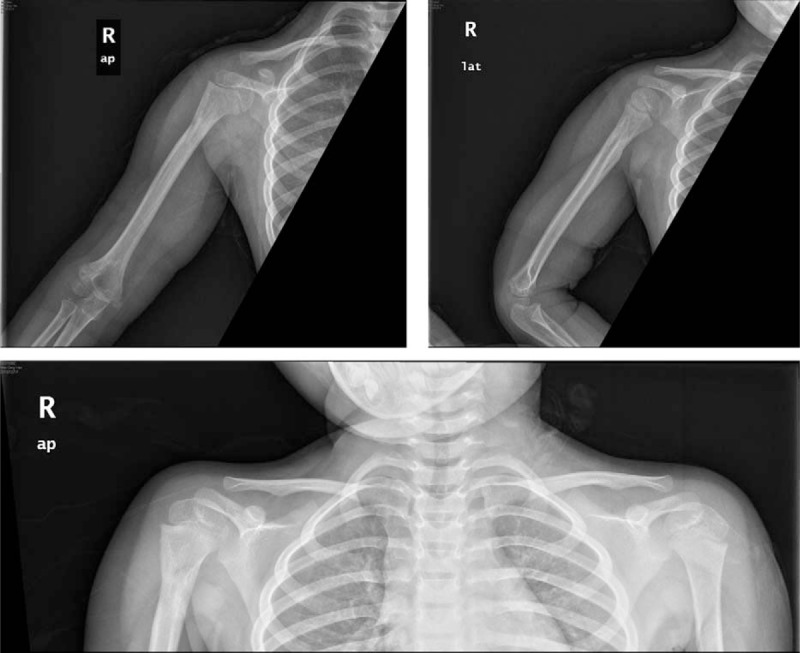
Anteroposterior and lateral x-ray and bilateral anteroposterior shoulders x-ray revealing complete fracture remodeling and no sign of avascular necrosis or any subsequent shoulder dislocation.
